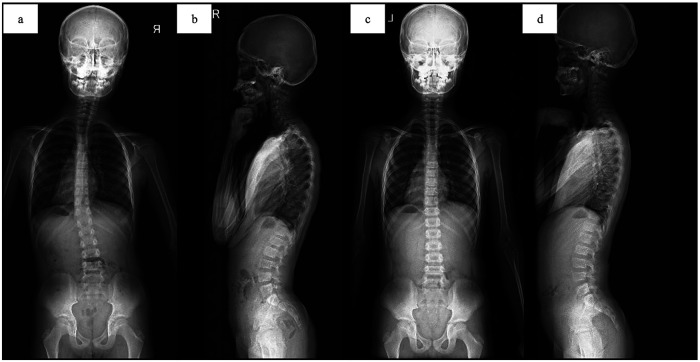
A typical case. The patient is a 10-year-old male participant, first diagnosed on July 28, 2023. x-Ray images show (a,b) the upper end vertebra at T7, the lower end vertebra at L3, with an initial Cobb angle of 15.32° and leftward scoliosis. The shoulder height difference was 19.69 mm, with the apex of the curve at T11, coronal balance offset at −18.59 mm, and vertebral rotation at grade 1. Sagittal balance was not recorded. Risser sign was grade 0, and the pelvic triangular cartilage state was grade 1. The patient underwent 4 sessions of rod-point treatment over a period of 1 month. Follow-up on September 1, 2023, showed (c,d) a Cobb angle reduced to 0.09°, shoulder height difference of 0 mm, coronal balance of 0 mm, and vertebral rotation remaining at grade 1.
